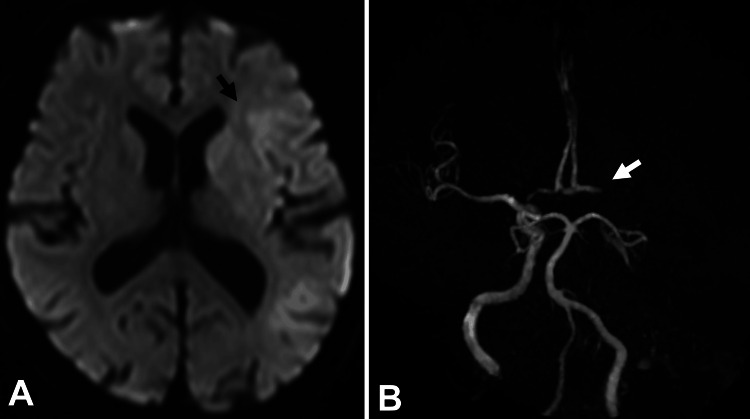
Preprocedural MRI and MRA. A. A diffusion-weighted MRI shows a faint high-intensity lesion in the area of the left ICA (black arrow). B. Cranial MRA revealing occlusion in the left ICA (white arrow). MRI, magnetic resonance imaging; MRA, magnetic resonance angiogram; ICA, internal carotid artery
